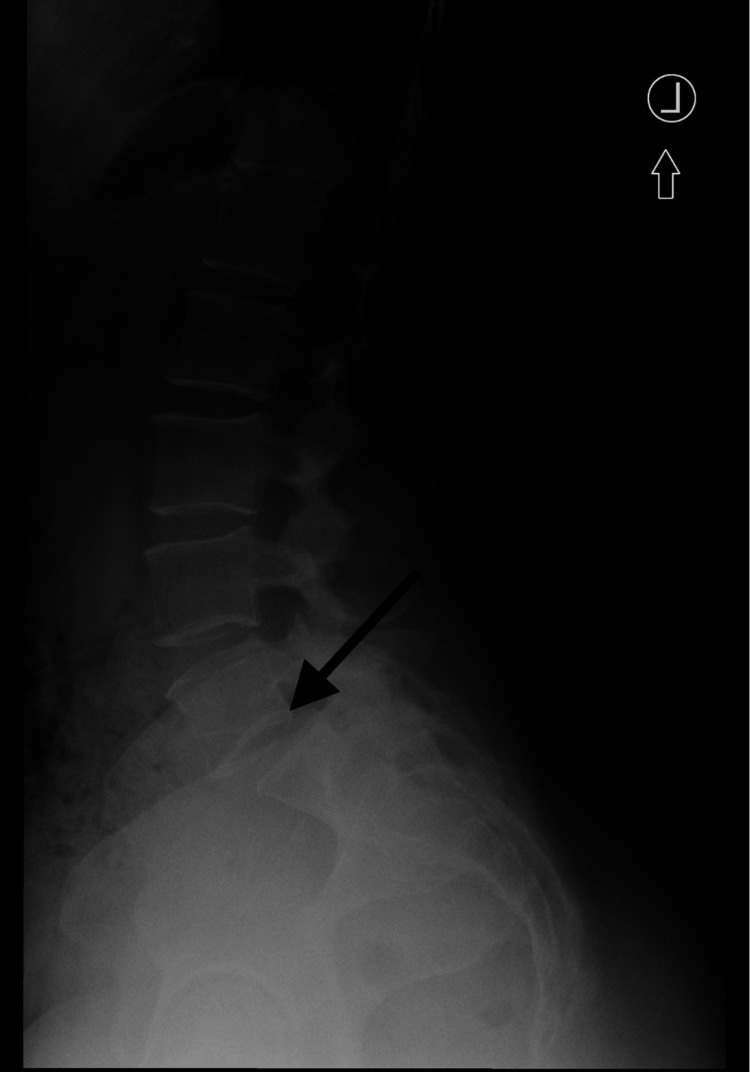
Lateral radiograph of the lumbar spine for Case 1. The black arrow indicates lumbar spondylolisthesis at L5-S1. L5: Lumbar Segment 5; S1: Sacral Segment 1.
